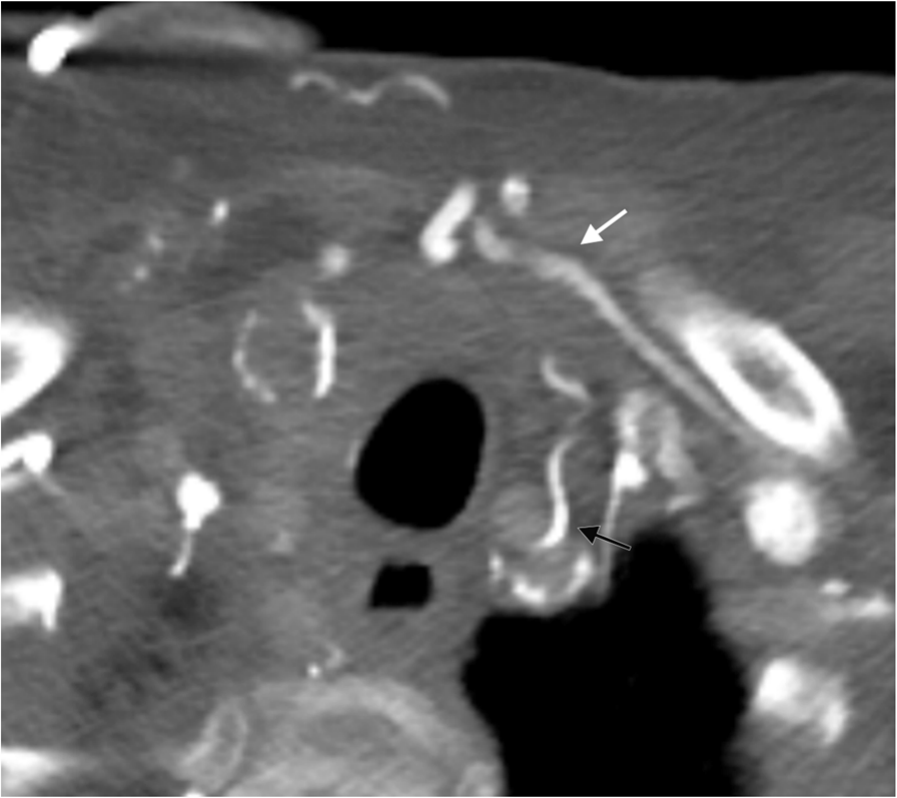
Anterior neck collaterals. Axial CT image showing the jugular venous arch (white arrow) assisting in the diversion of blood flow to the patent contralateral side and an enlarged inferior thyroid vein collateral (black arrow) in a patient with an obstructed internal jugular vein
